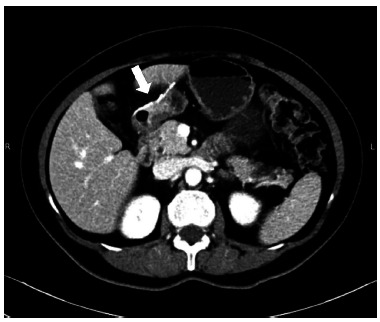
Postoperative axial image of abdominopelvic CT (arrow=tumor resected)
